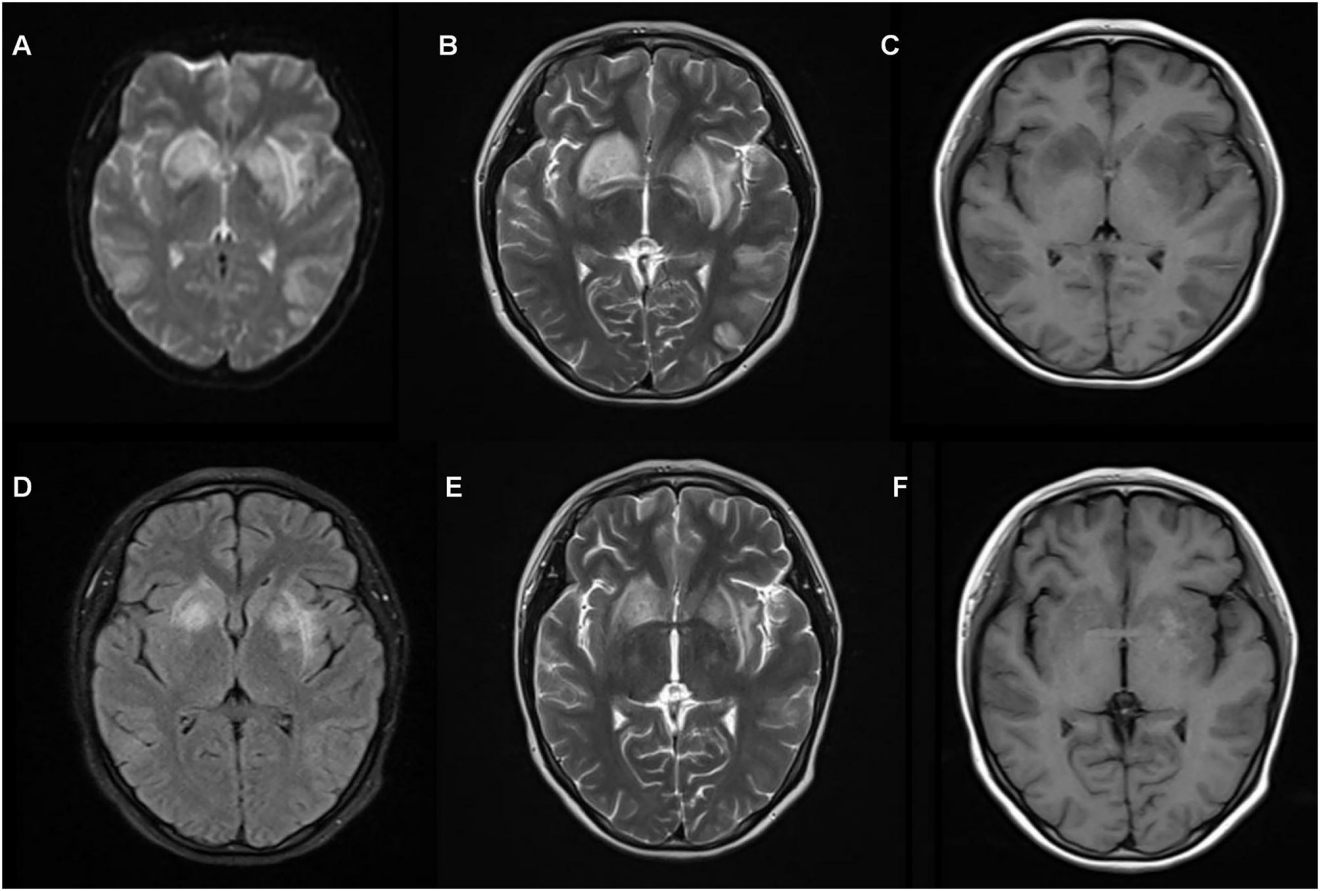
Magnetic resonance imaging (MRI) of case 2 (A–C) shows a wide range of abnormal signals in both hemispheres and basal ganglia region before pulse therapy. Follow-up brain MRI (D–F) after 15 days shows normal results.
